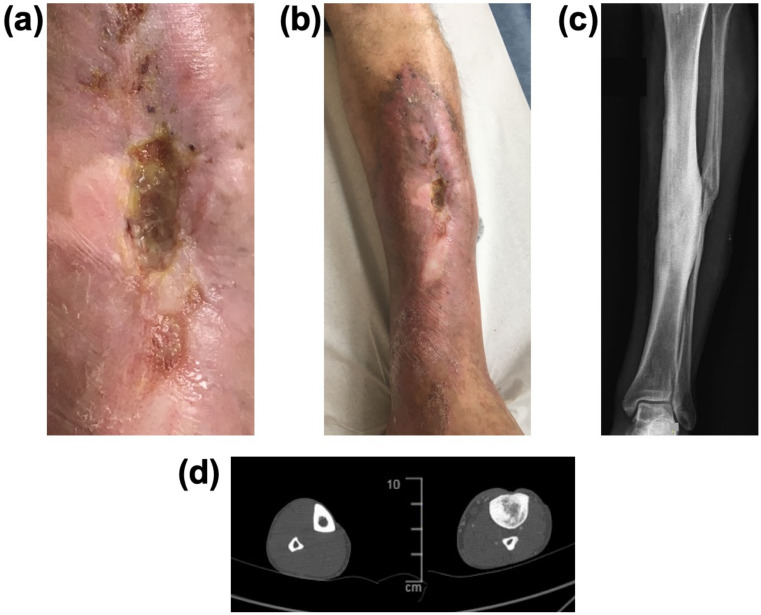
Post-traumatic left tibial chronic osteomyelitis in a French patient in failure of phage treatment administered in Georgia. A ready-made cocktail was administered locally and *per os* for several weeks. Bone exposure persisted (a) with large skin adherences (b) to underlying sclerotic bone (c) X-ray without any abscess or intramedullary cavity on CT-scan (d); transverse view of both legs; on the left the leg without infection, on the right the infected leg, with sclerotic bone and densification of the medulla). Heavy high-tech surgery in a CRIOAc reference center is preferably indicated, with bone curettage (only means of eradicating infected necrotic bone), resection of the large skin area around the exposed bone, related to chronic inflammation and unable to recover, with free-flap cover followed by prolonged antimicrobial therapy. Intraoperative and postoperative phage administration is clearly not feasible in such a patient.
